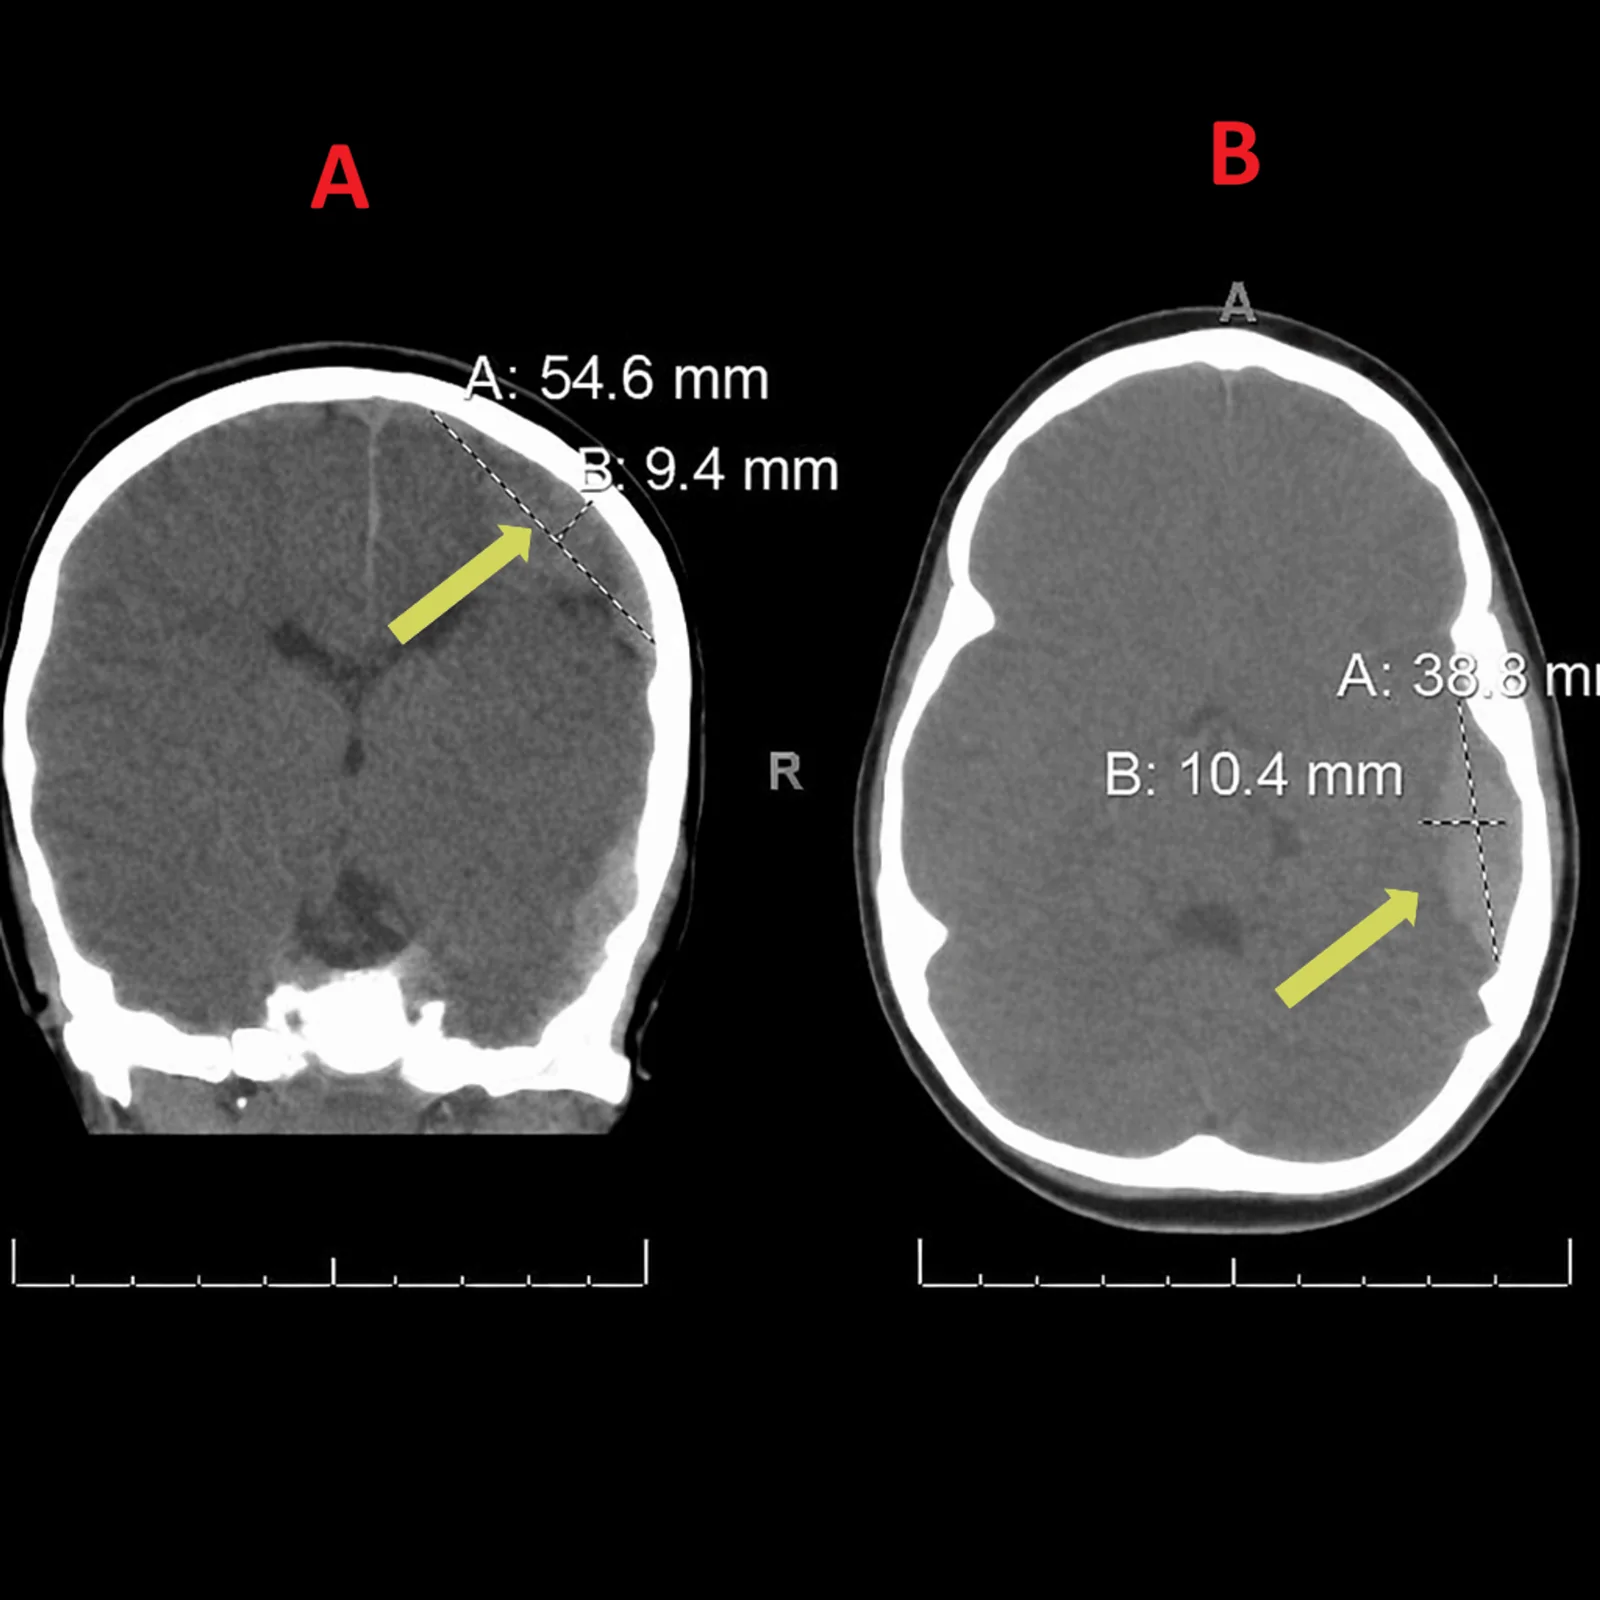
Noncontrast CT images of the brain demonstrating left-sided extra-axial hemorrhage. (A) Coronal CT image demonstrating a left high parietal subdural hematoma measuring approximately 5.4×0.9 cm, with mixed densities suggestive of a subacute component (arrow). An underlying left parietal intraparenchymal hemorrhagic focus measuring approximately 18×18 mm is also noted. (B) Axial CT image showing a left temporal acute epidural hematoma with a lentiform configuration measuring approximately 3.8×1.0 cm (arrow). The ventricular system is normal in size and configuration, with no evidence of midline shift or significant mass effect. The midbrain and posterior fossa structures appear unremarkable.
